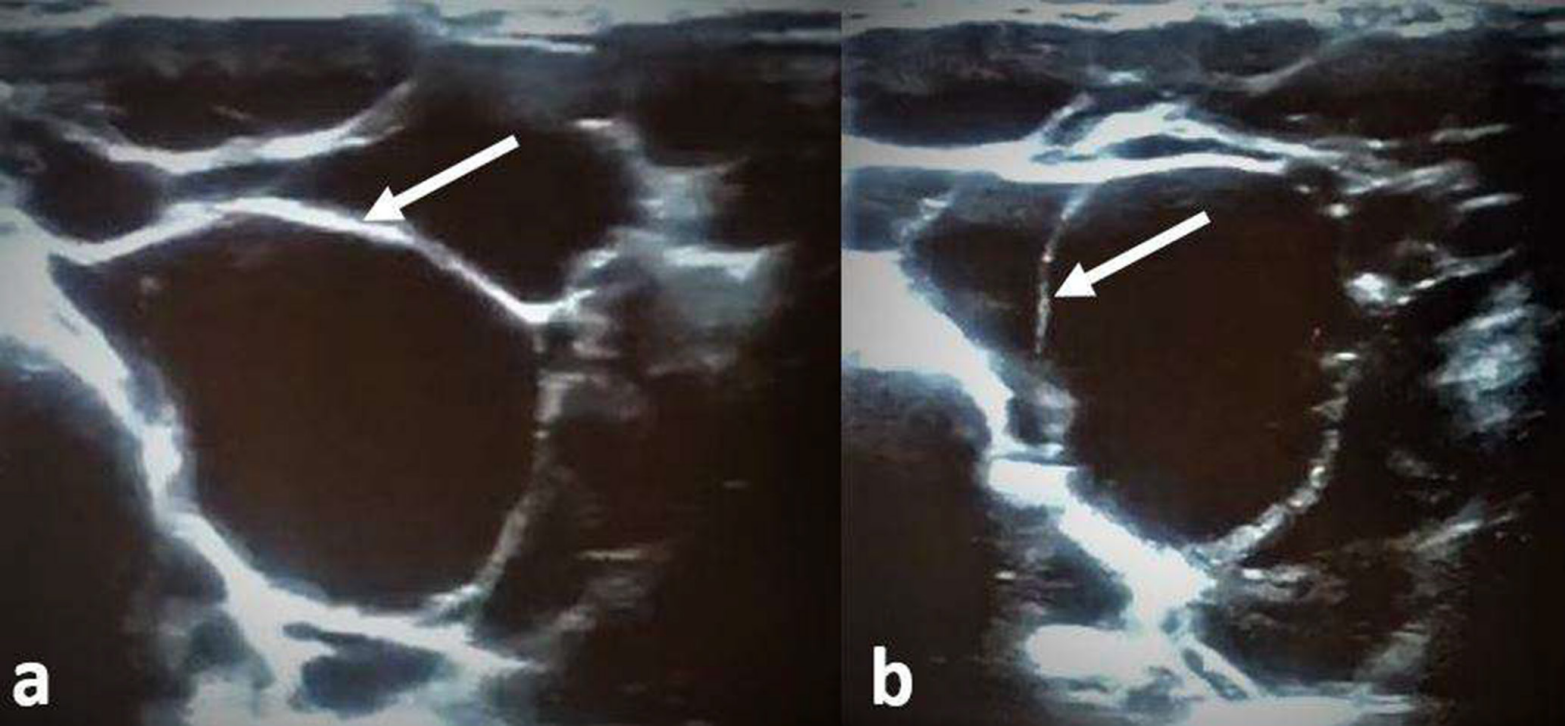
(a, b) Abdominal ultrasound showing a multiloculated cyst in the right iliac fossa containing a few thin septa (white arrows) without vascularisation in Doppler.
